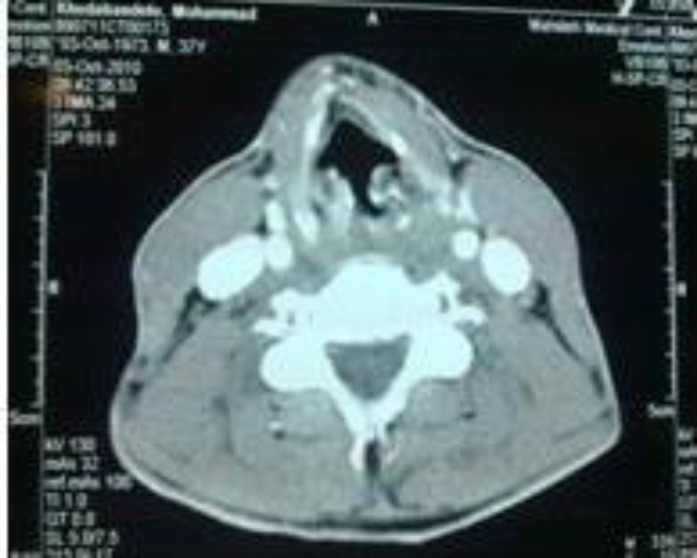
CT scan with contrast but without significant enhancement
